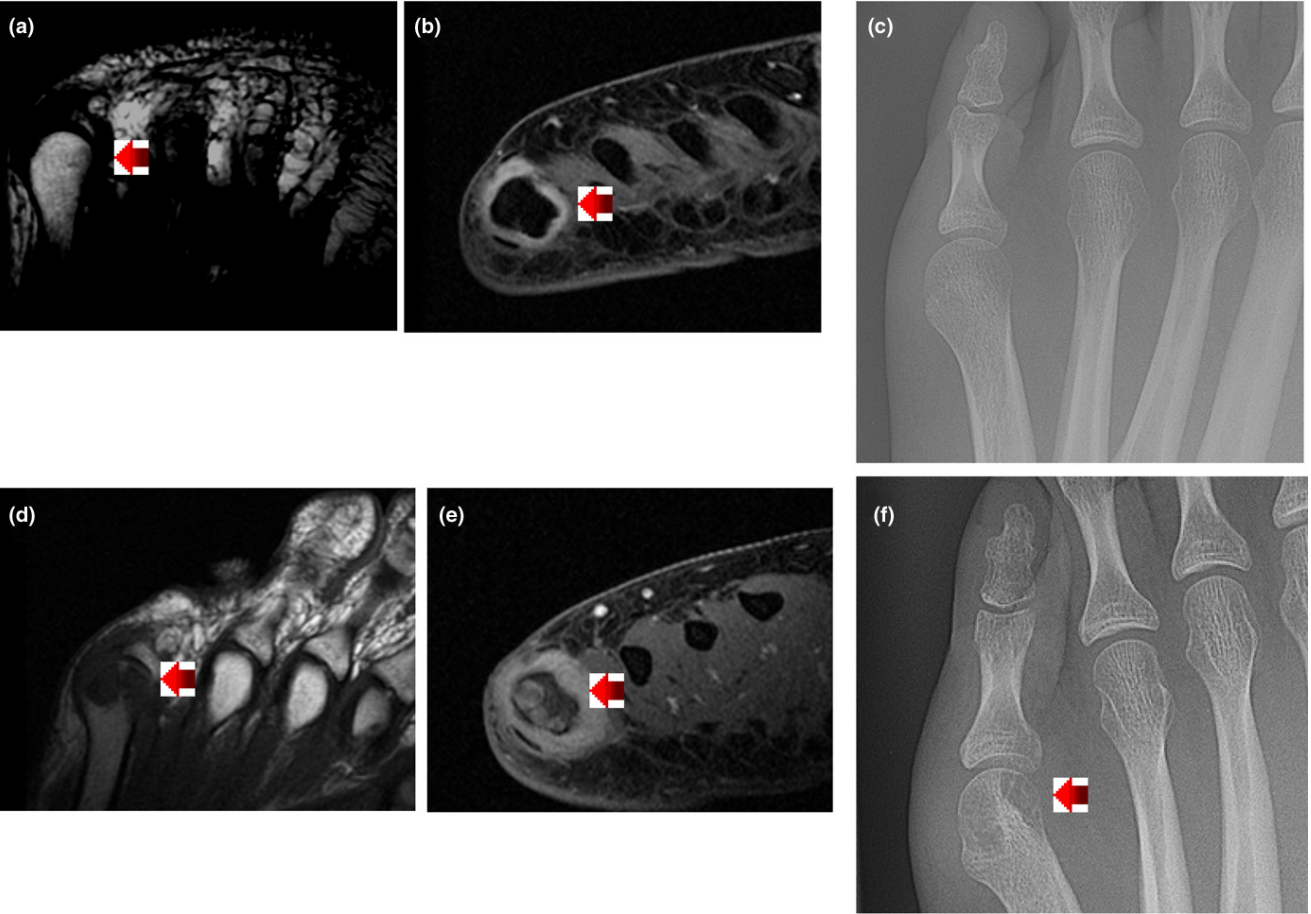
Sample imaging depicting bone defect on magnetic resonance imaging before radiographic erosion. Images (a-c) were taken 1 year before images (d-f). Image (a) is T1-weighted and has a small defect (arrow). Image (b) is T2-weighted with fat saturation clearly showing synovitis (arrow). Image (c), the radiograph, was read as normal because the cortex was intact. Image (d), T1-weighted, clearly shows absence of bone read as erosion (arrow). Image (e) shows synovitis and bone marrow edema (arrow). Image (f) clearly shows bone erosion (arrow).
